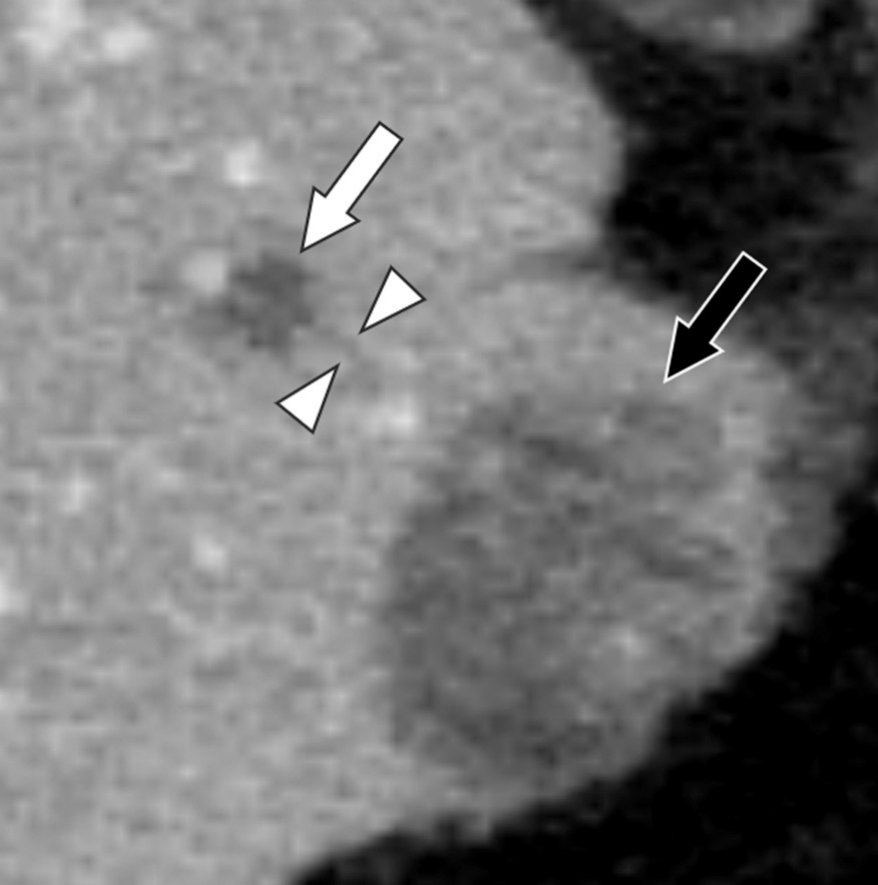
Axial contrast-enhanced CT 2 days after cryoablation reveals a small portal venous thrombus (arrowheads) adjacent to the subsegmental portal venous thrombus (white arrow) toward the ablation zone. There is no continuity between the small thrombus and the ablation zone (black arrow).
